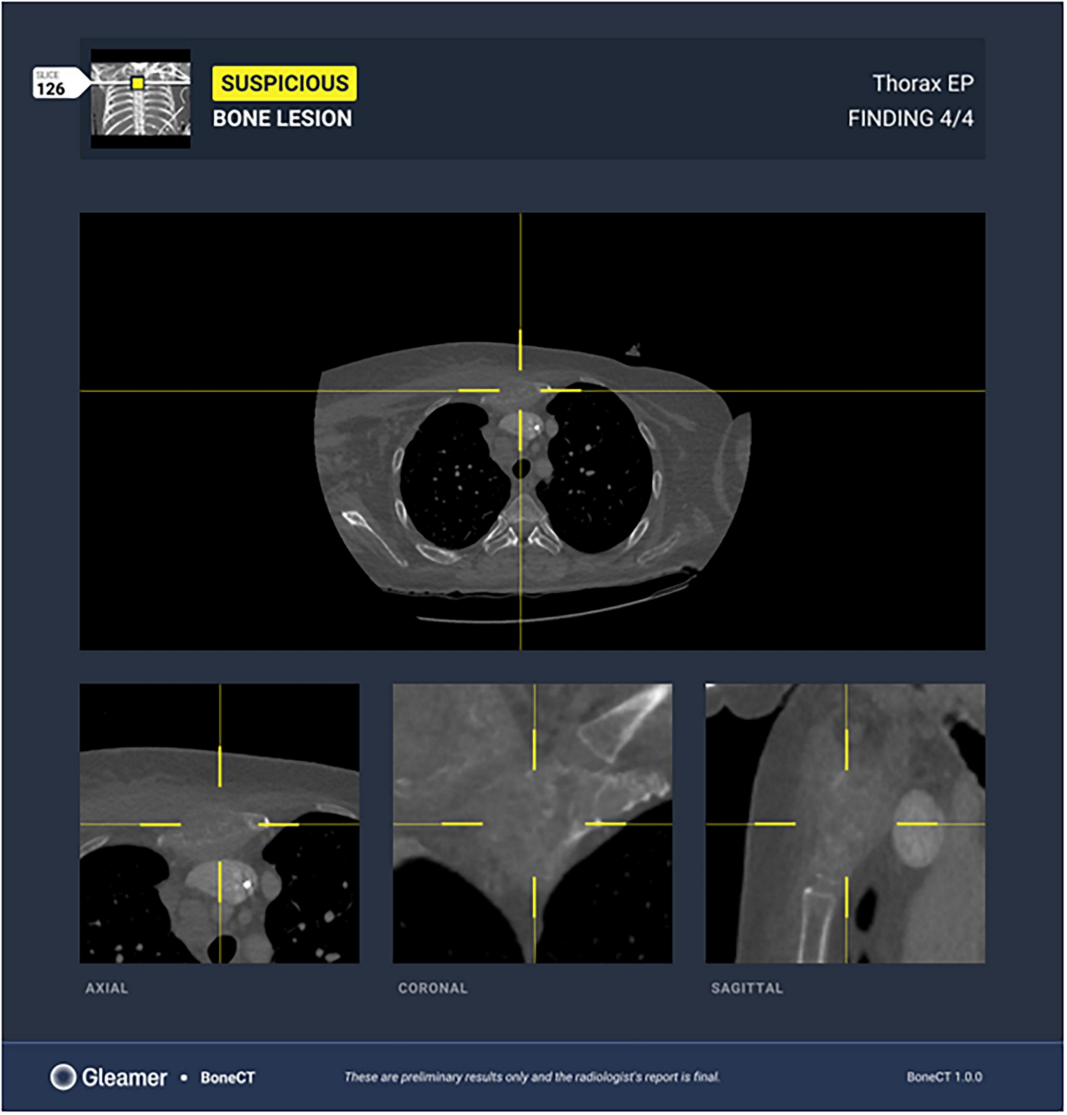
Detection of lytic focal bone lesion of the sternum on CT-scanner with GLEAMER BoneCT software.
